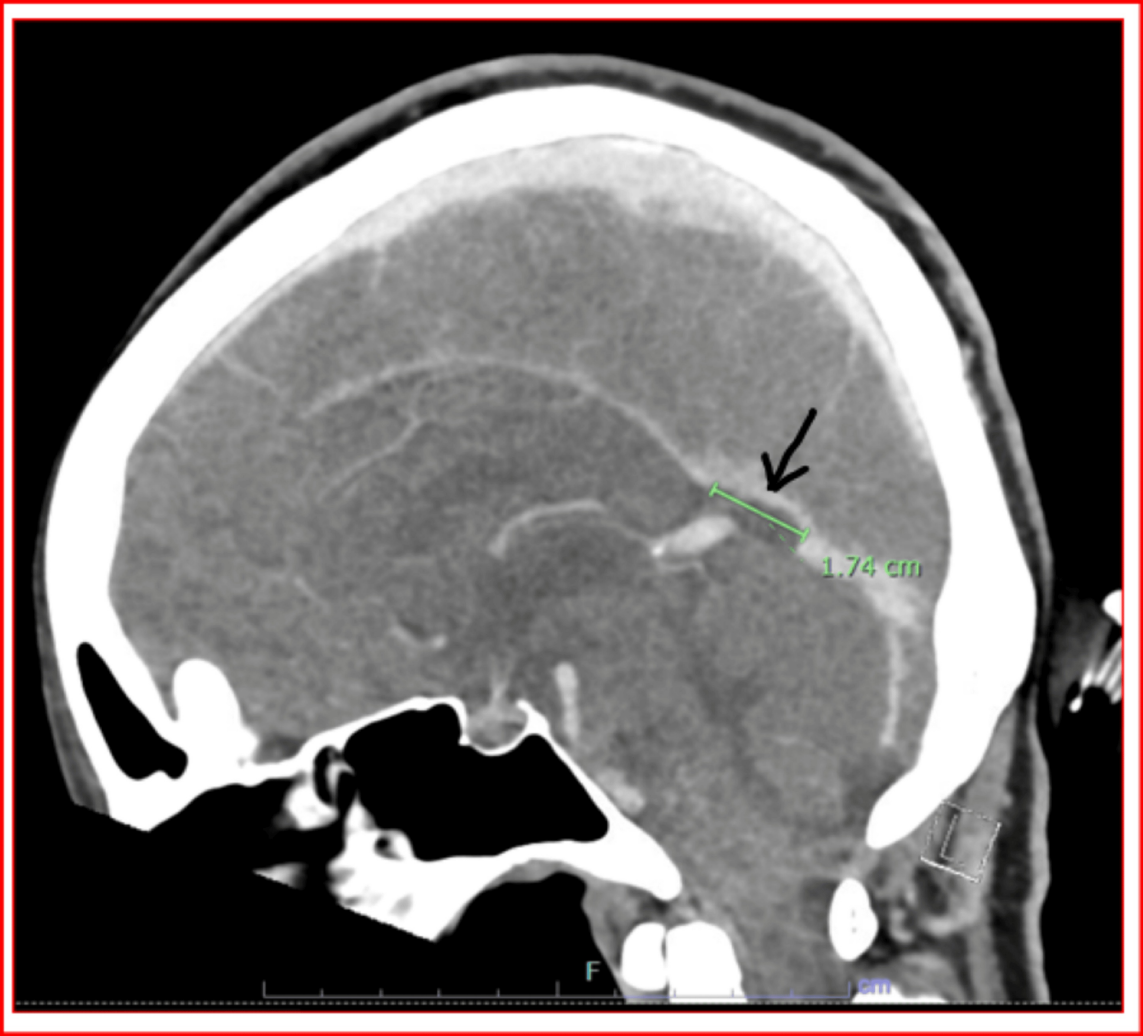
CT venogram showing focal filling defect up to 1.7 cm at the level of the proximal straight sinus, suggestive of thrombus formation and normal venous return in distal straight sinus with a visible right internal jugular vein
